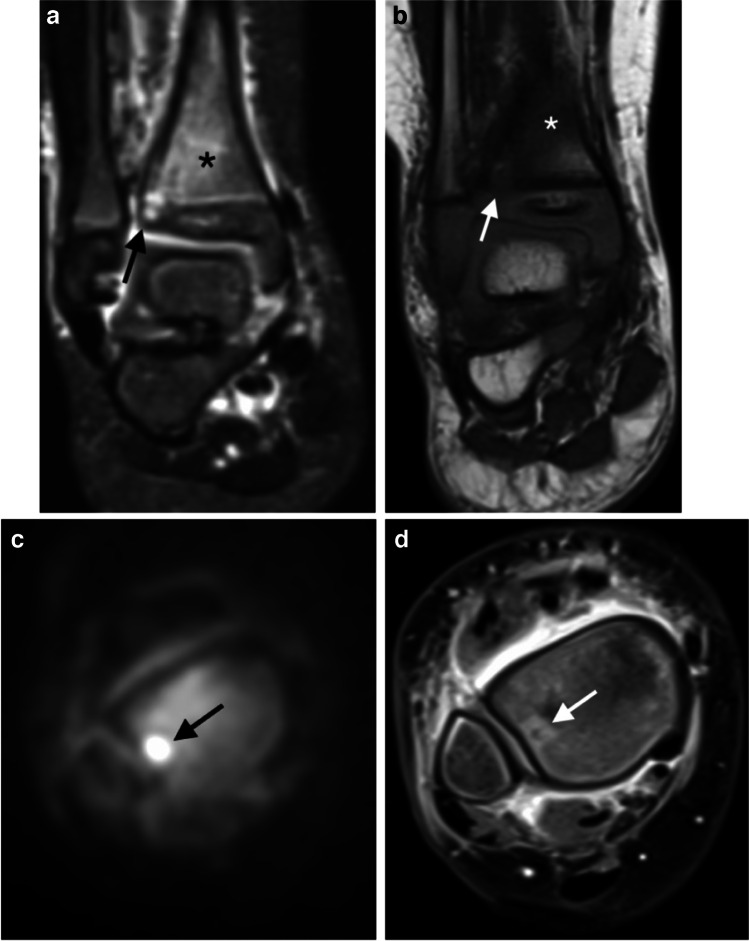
A 20-month-old boy with bone abscess in osteomyelitis of the distal tibia. a Coronal STIR shows a focus of high signal of the metaphysis and physis of the distal tibia (*arrow*), within a background of medullary hyperintensity, consistent with osteomyelitis (*star*). b A coronal T1-weighted image shows corresponding focal hypointense signal (*arrow*) and diffuse hypointense metaphysis (*star*). c Axial DWI (b value of 800 s/mm^2^) depicts a focus of hyperintensity (*arrow*). d An axial T1-weighted fat-suppressed image after contrast injection confirms a small bone abscess with peripheral enhancement around a low signal core (*arrow*). DWI diffusion-weighted imaging, STIR short tau inversion recovery
